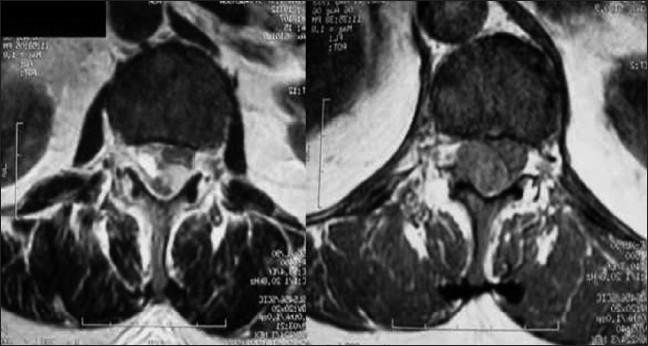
Axial MR image showing the biconvex nature of the lesion
